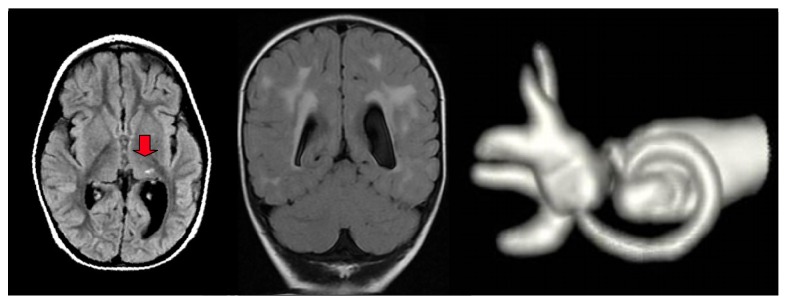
MRI scans, after effects of CMV meningoencephalitis, with patchy lesions of the white matter (red arrow) and dilation of the left lateral ventricle. 3D MR image of a case of semicircular canal occlusion, complication of CMV meningoencephalitis.
